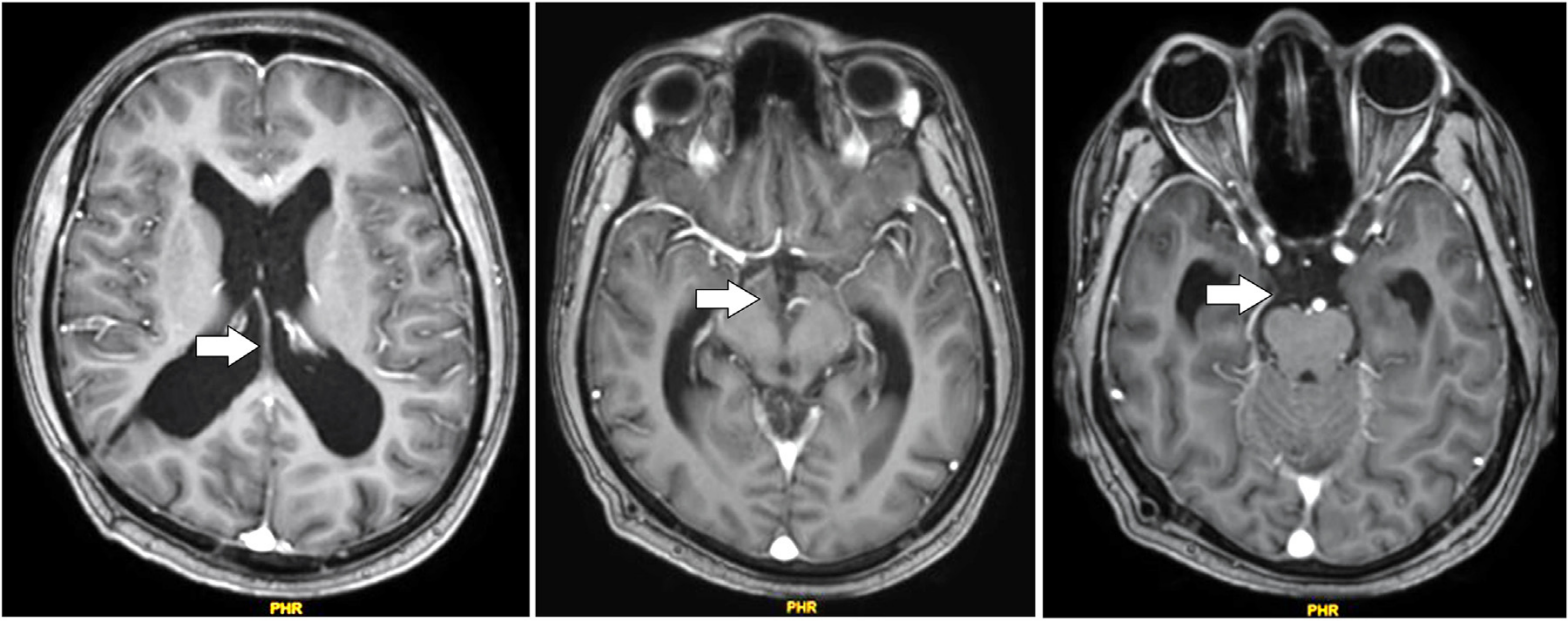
First follow up T1 contrast MRI axial images after completion of radiotherapy showing complete response with no residual tumor in the pineal region.
